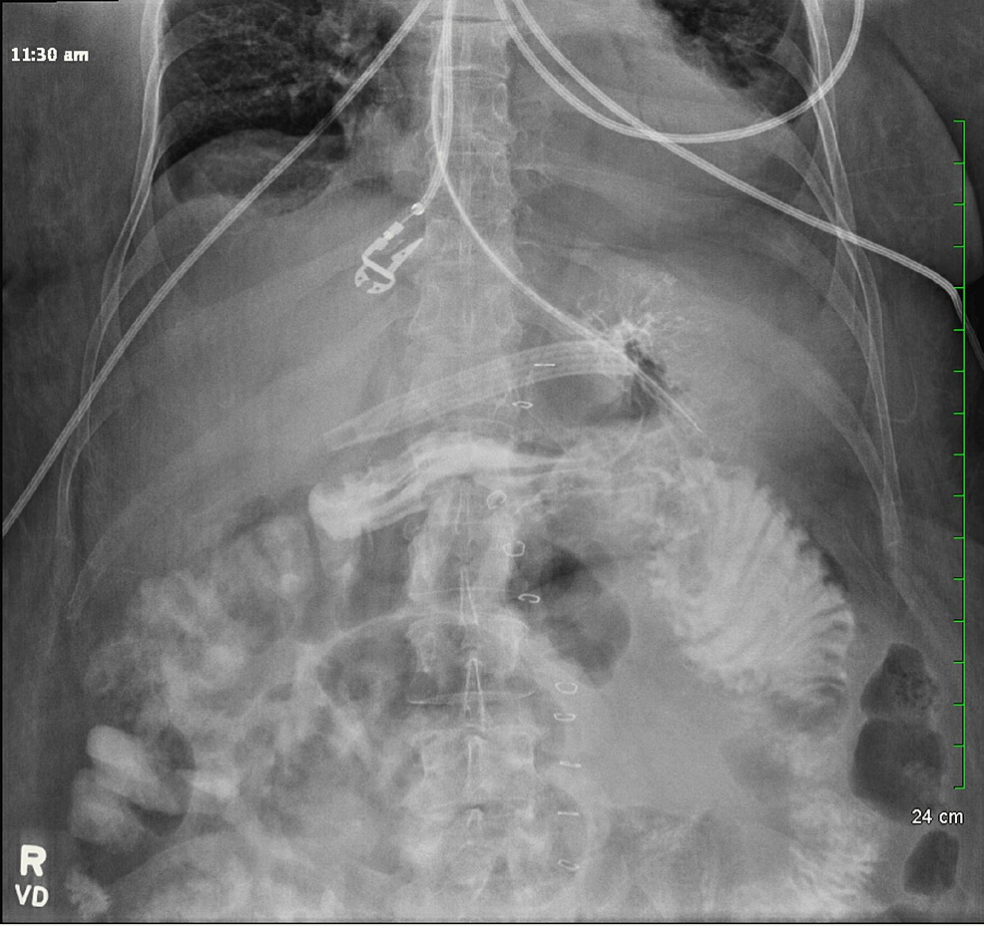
Upper gastrointestinal tract radiography with fluoroscopy showing contrast opacifying the stomach and flowing to the gastrojejunostomy without obstruction and extravasation.
